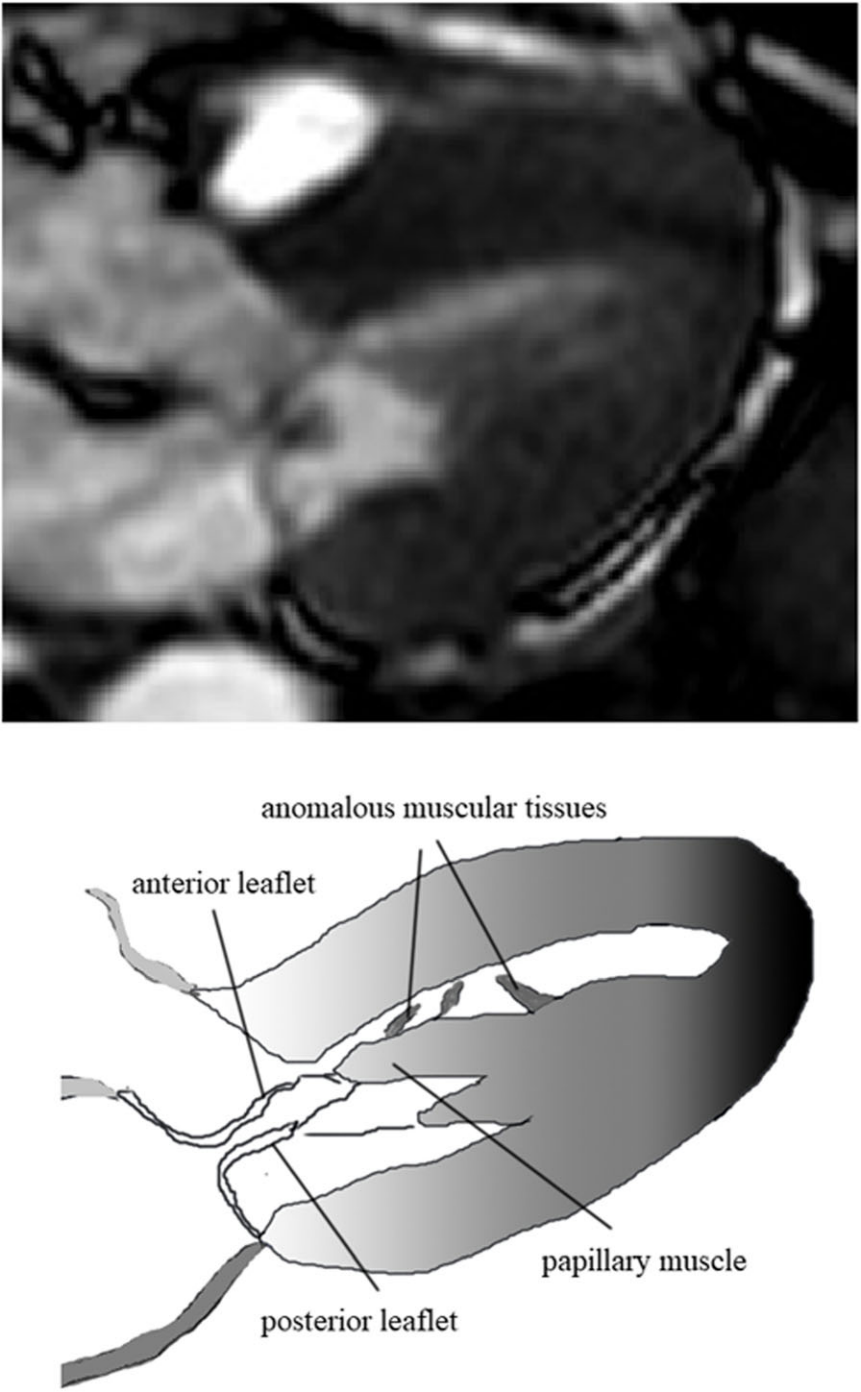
Cardiac magnetic resonance imaging in late systole. In this patient, two mechanisms of SAM were postulated. One was that the tips of papillary muscles anomalously deviated anteriorly. The papillary muscles were connected with the septum with abnormal fibrous tissue and touching the septum in systole. The other mechanism involves the elongated anterior leaflet. It was turned over by the blood flow through LVOT, as described by Schwammenthal et al. [1]
